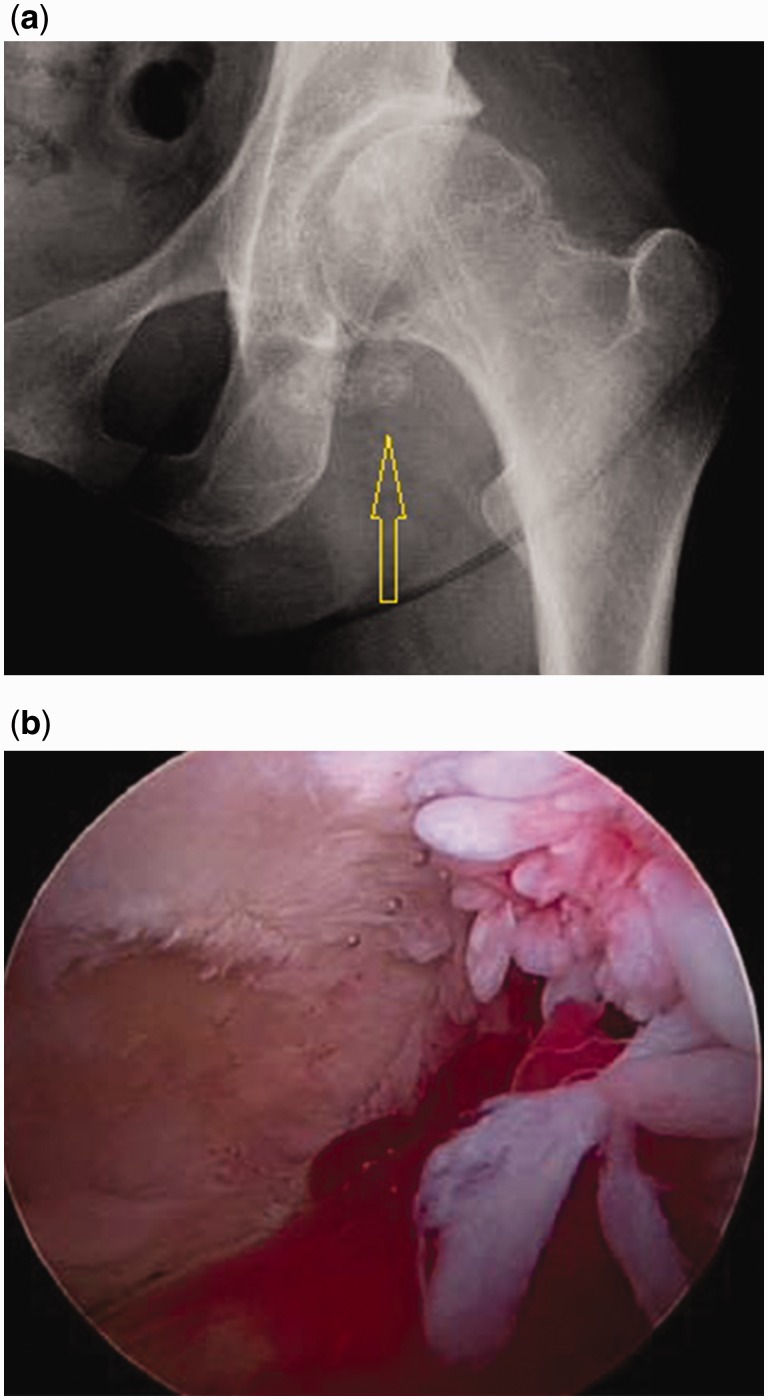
(a) Anteroposterior hip radiograph with visible loose bodies(arrow). (b) Intraoperative photo showing inflamed synovium and degenerative changes in the joint.
